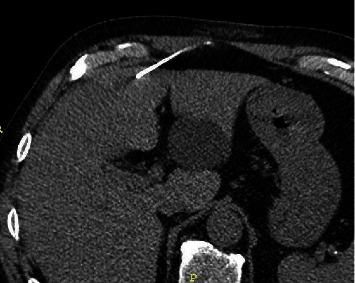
CT guided core biopsy of segment 4 hepatic lesion.
